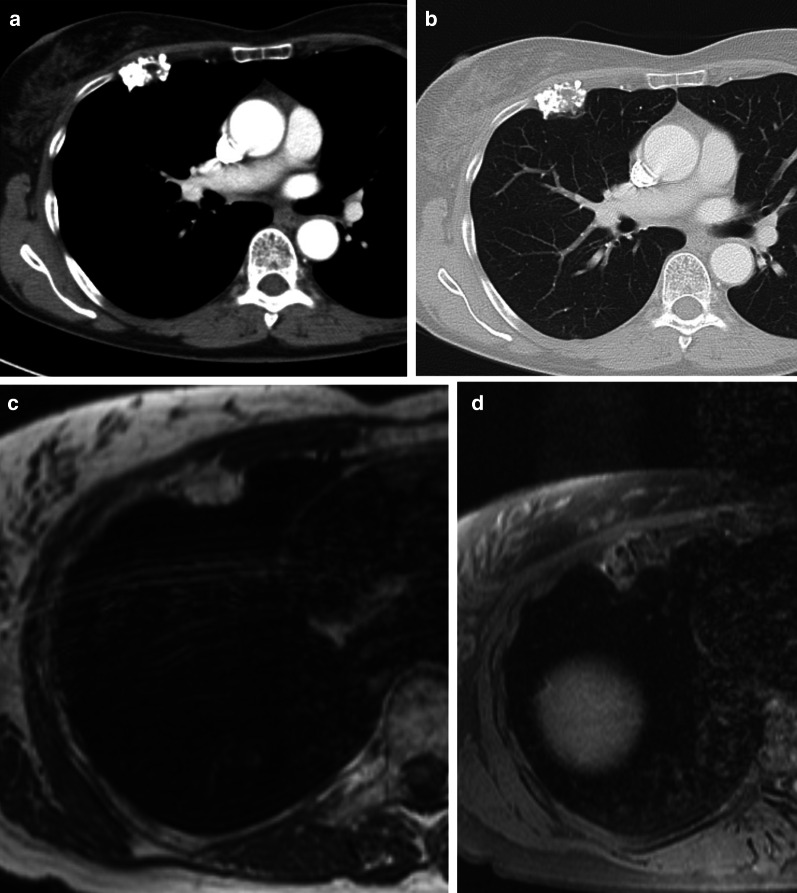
Diagnosis: chondrosarcoma. Technique: contrast-enhanced chest CT and MRI. Description: A 64-year-old woman with a history of smoking, presented due to shortness of breath. The axial contrast-enhanced CT scan (a, b) reveals an expansile lesion with extensive calcifications located at the costochondral junction of the right 3rd rib (note a typical arc-and-ring pattern of calcification). On the axial T1-weighted MR image (c) the lesions have a high signal intensity. The axial T2-weighted MR image (d) shows a peripheral hyperintense mass with internal low-intensity foci, reflecting the cartilaginous matrix with chondroid calcifications
